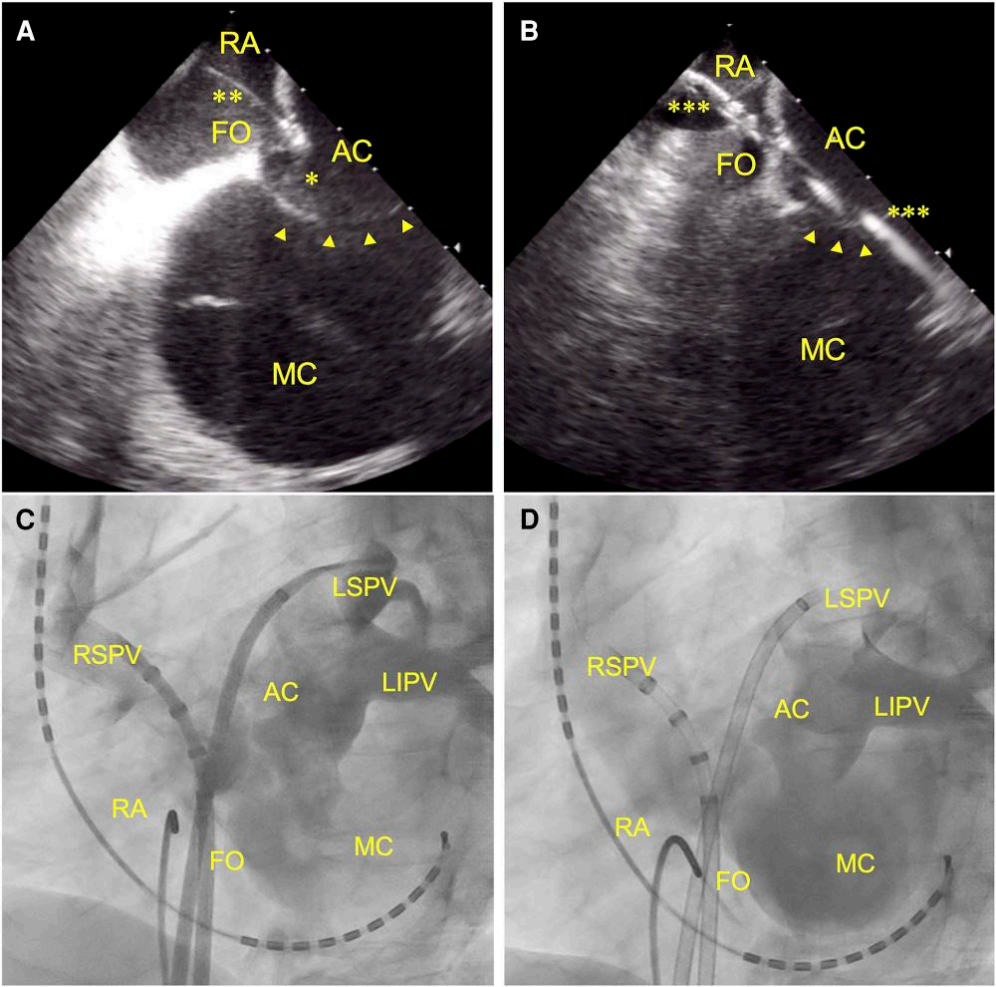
Transseptal puncture with intracardiac echocardiography (*A* and *B*) and pulmonary venography (*C* and *D*). (*A*) Before transseptal puncture using a transseptal needle (). The needle tip (*) is pushing the fossa ovalis towards the accessory chamber. (*B*) After transseptal puncture. Guidewires (*) are inserted into the accessory chamber through the fossa ovalis. (*C* and *D*) Confirmation of precise catheterization into the accessory chamber with contrast infusion. AC, accessory chamber; MC, main chamber; FO, fossa ovalis; RA, right atrium; RSPV, right superior pulmonary vein; LSPV, left superior pulmonary vein; LIPV, left inferior pulmonary vein. Arrow heads, membrane.
